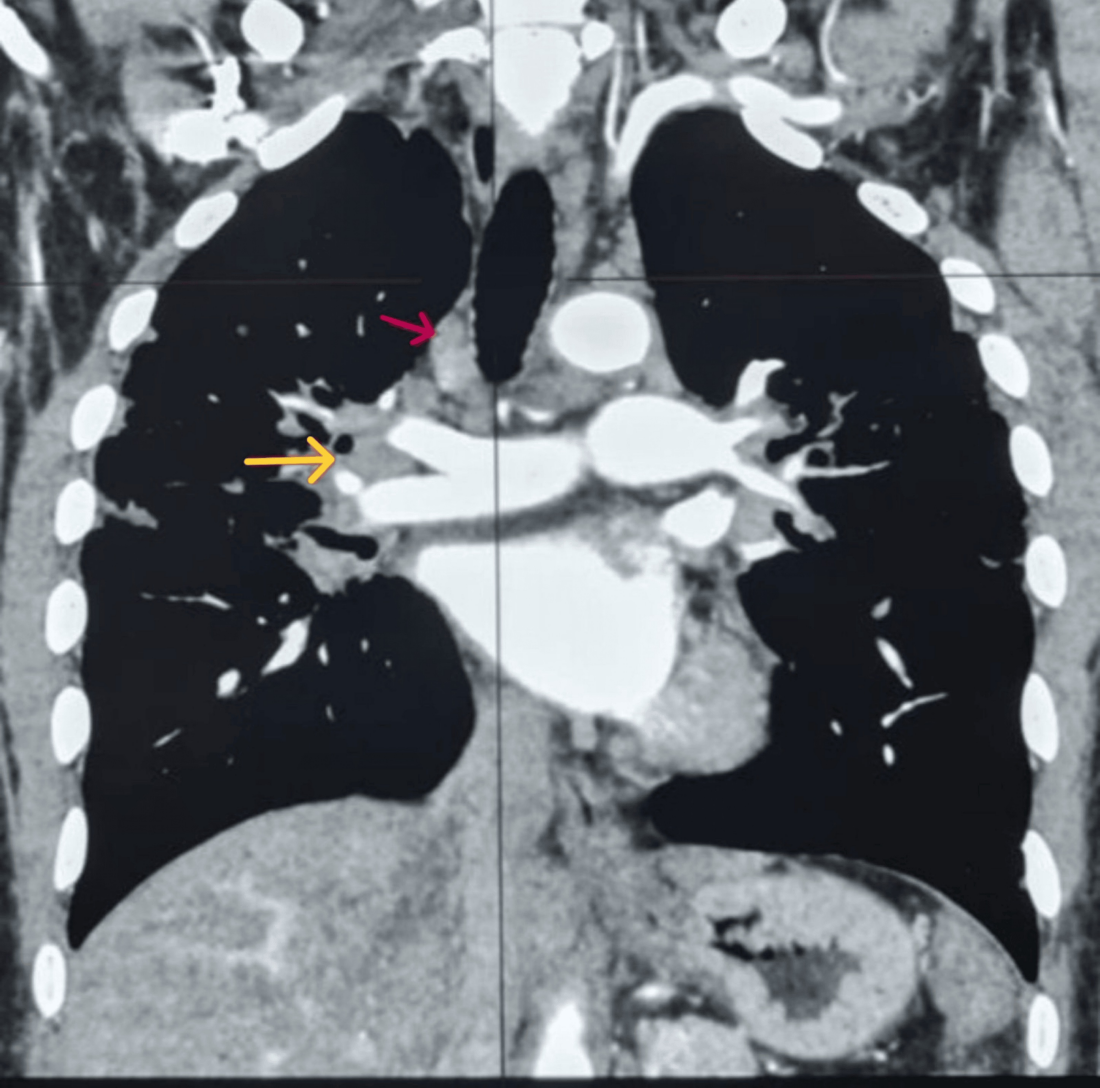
CT chest (coronal reconstruction, mediastinal window, contrast-enhanced) demonstrating anterior superior mediastinal lymphadenopathy (red arrow) and right hilar lymphadenopathy (yellow arrow).
